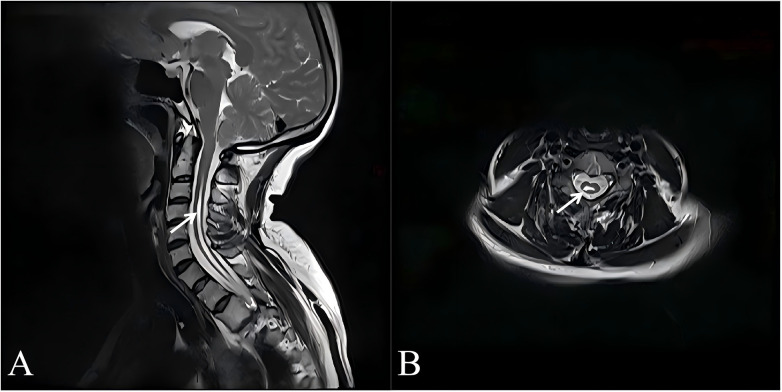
Preoperative cervical spine MRI. Sagittal view (A) and axial view (B). The white arrows in A and B indicate the syrinx cavity.
